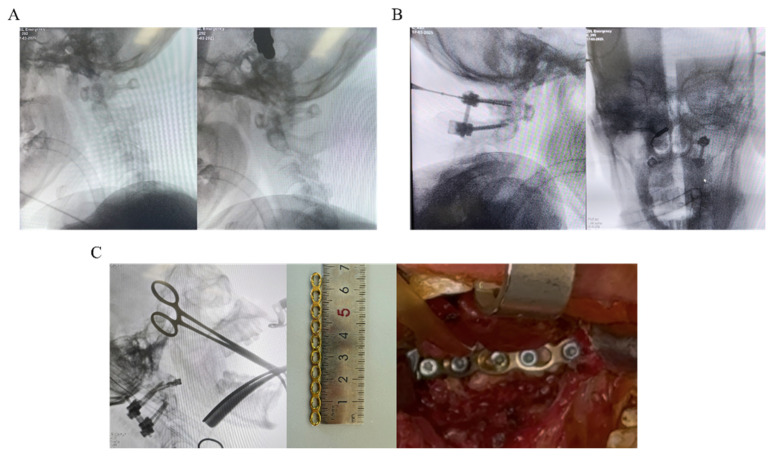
Intraoperative imaging examinations: (A) Lateral cervical radiographs acquired before and after skull traction. (B) Lateral cervical radiograph during posterior internal fixation. (C) Anterior approach intraoperative imaging.
